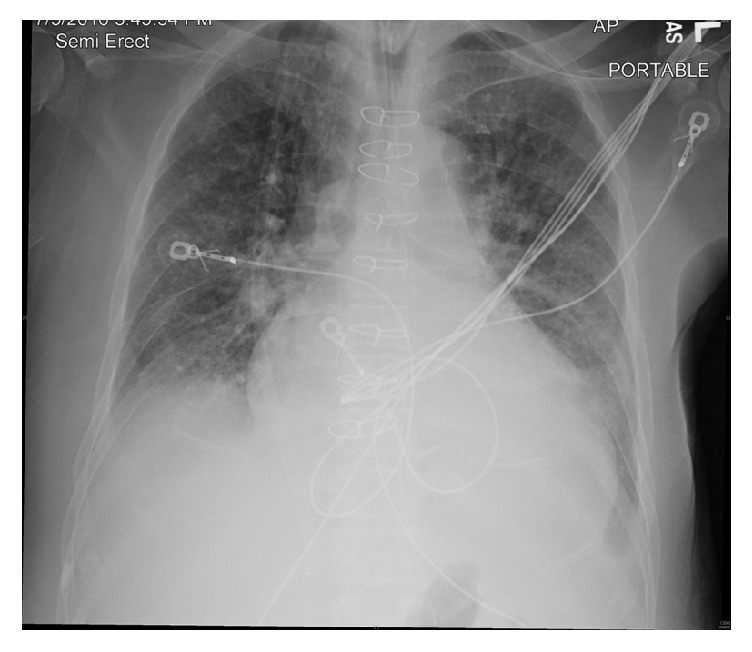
Portable chest radiograph following right-sided thoracentesis showing significant reduction in pleural effusion.
